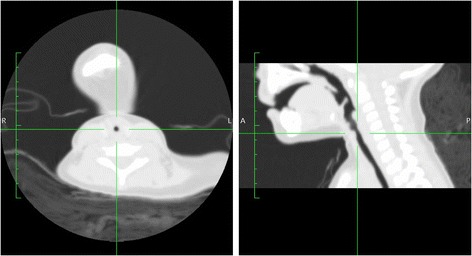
Neck CT images taken at POD 7. Tracheal stenosis was improved but still existed at the subglottic level. The sagittal plane image demonstrates the process of the anterior wall of the trachea (*arrow* in the *right panel*). *Left*, transverse image of the neck. *Right*, sagittal image of the neck
